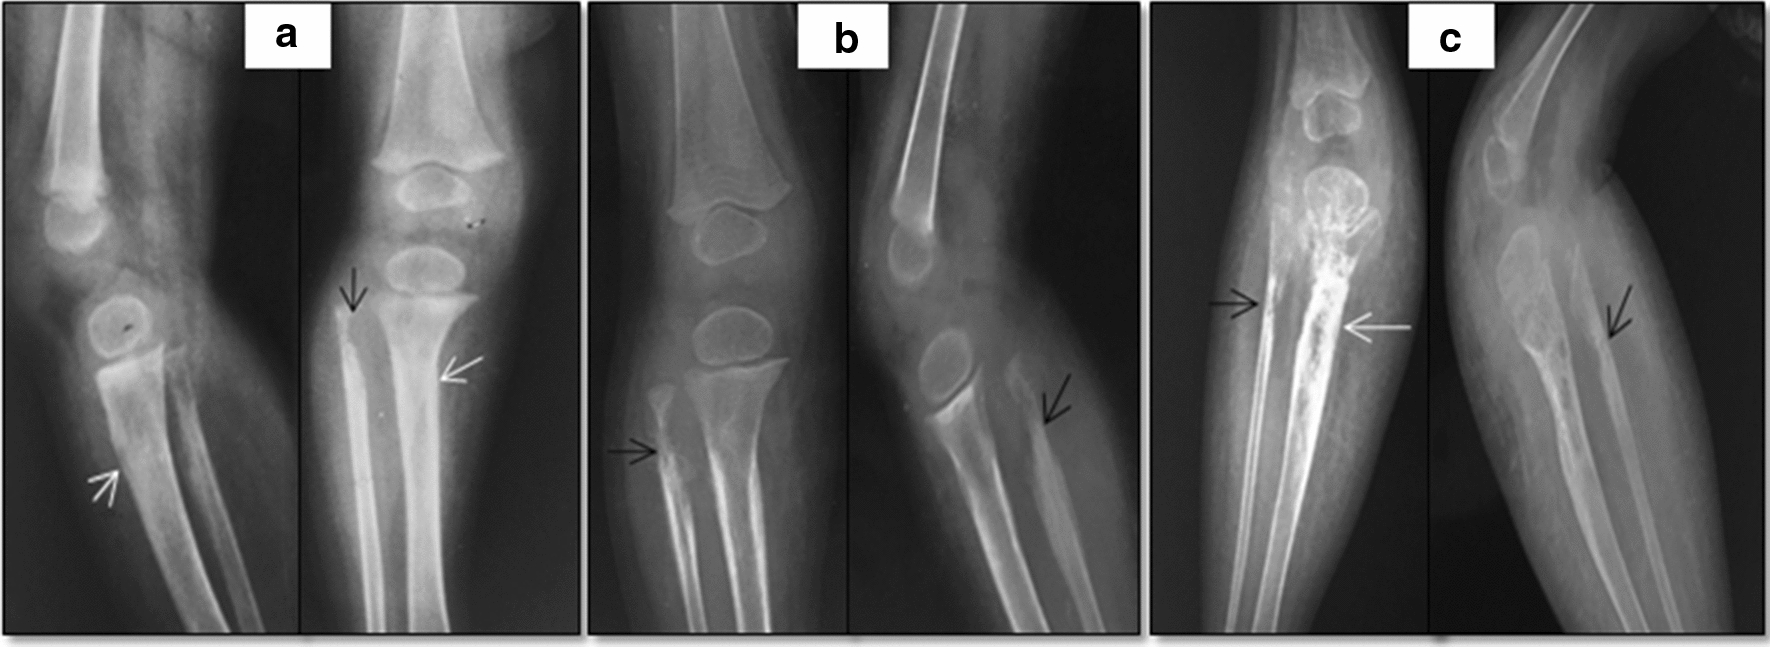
Sequential radiographs showing soft tissue and osseous changes in tibia (white arrows) and fibula (black arrows) over span of two years. Initial X-ray of 2016 a shows ill-defined sclerosis in proximal tibia and cortical erosion along the medial cortex of fibula which further progresses to circumferential cortical erosion in follow up radiograph of 2017 (b). The X-ray following three surgical debridements c demonstrates mixed lytic sclerotic changes involving proximal tibia and fibula. Gradually progressive soft tissue swelling is seen with predominant fat proliferation involving calf of right leg
